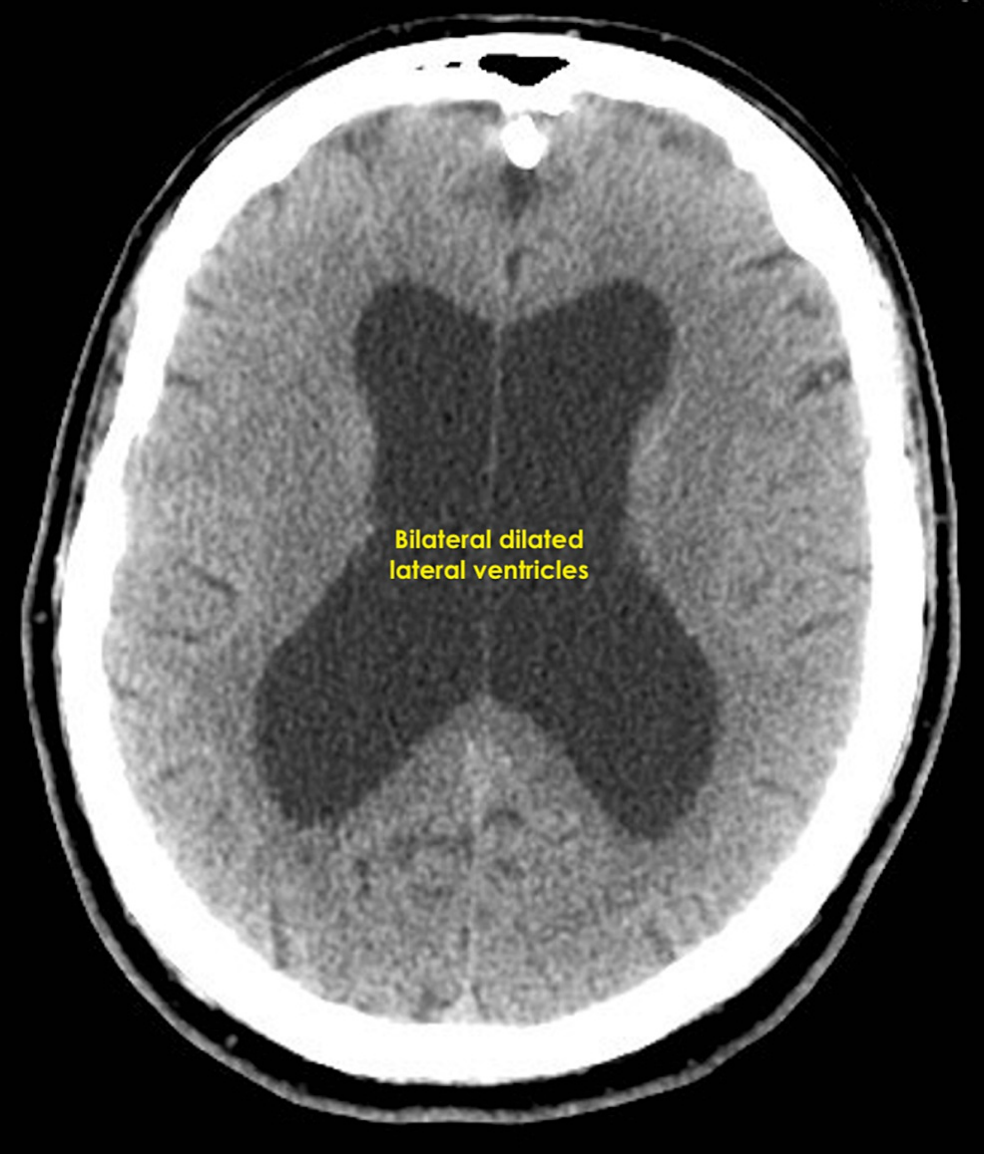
Axial brain computed tomography scan demonstrating bilateral dilated lateral ventricles typical of hydrocephalus
